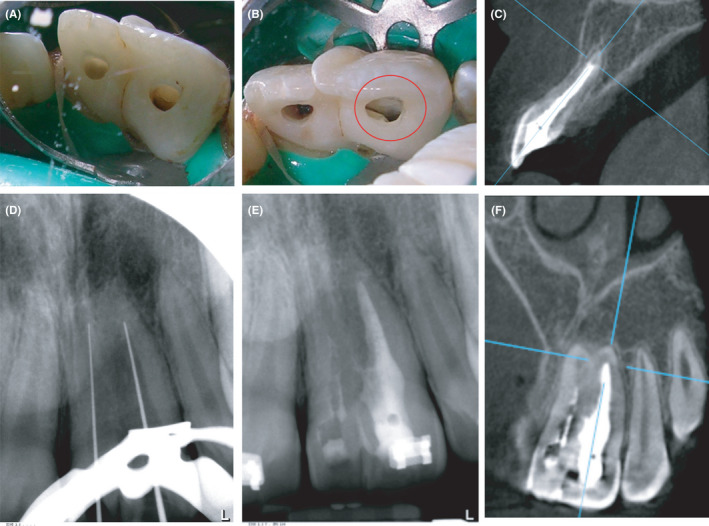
Root canal treatment of double‐tooth prior to surgical sectioning procedures. (A) access cavity preparations in the maxillary left central incisor and fused supernumerary tooth; (B) image showing communicating isthmus between maxillary left central incisor and conjoined supernumerary tooth; (C) showing length of mineral trioxide aggregate (MTA) monoblock filling; (D) working length measurement for the fused tooth; (E) immediate post‐filling periapical radiograph showing MTA monoblock filling. Note the presence of communicating fins between the two teeth; (F) Coronal CBCT slice showing the MTA monoblock filling
